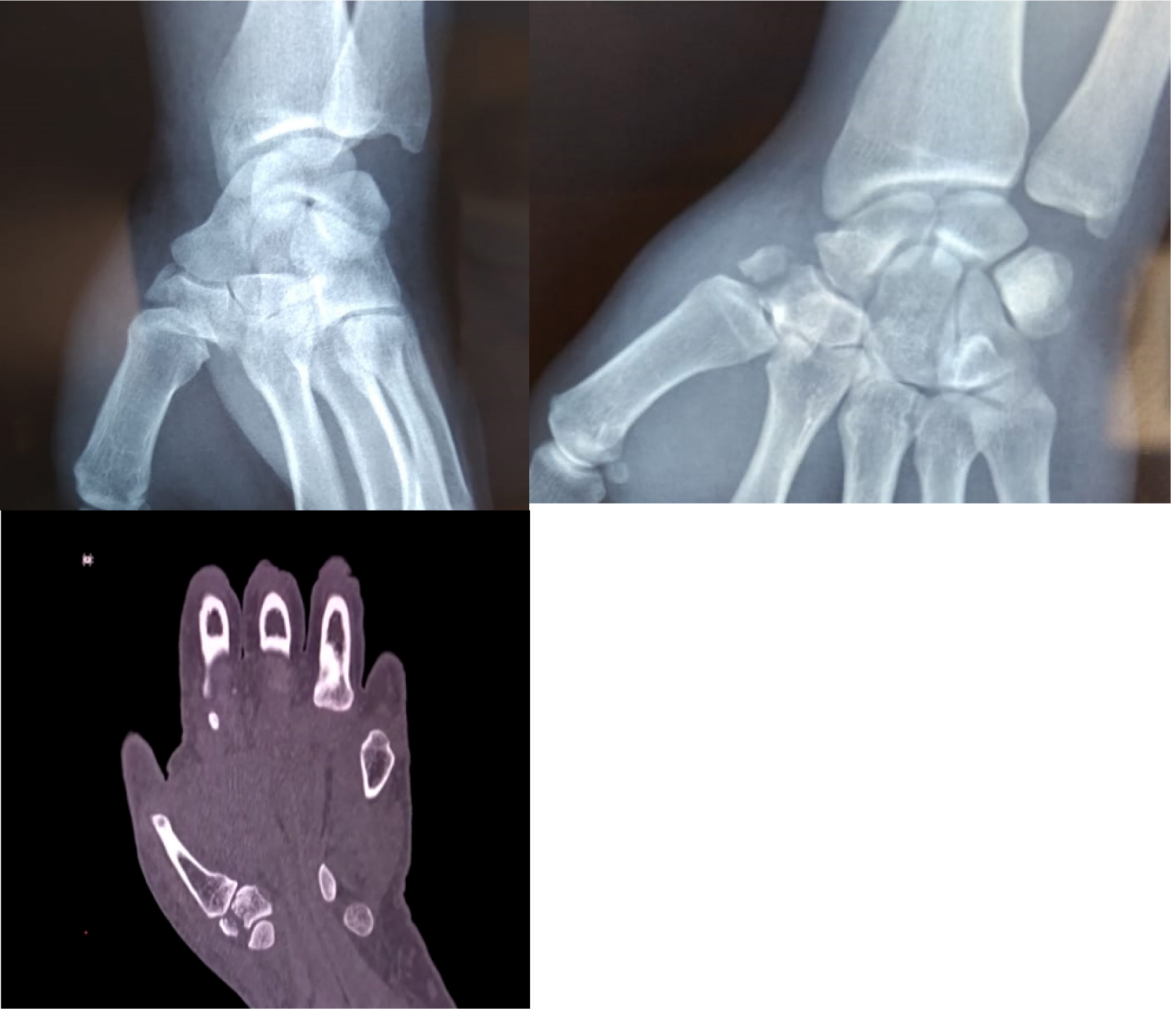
Standard x‐ray and CT scan showing the fracture of the body of the trapezium bone with a vertical line.
